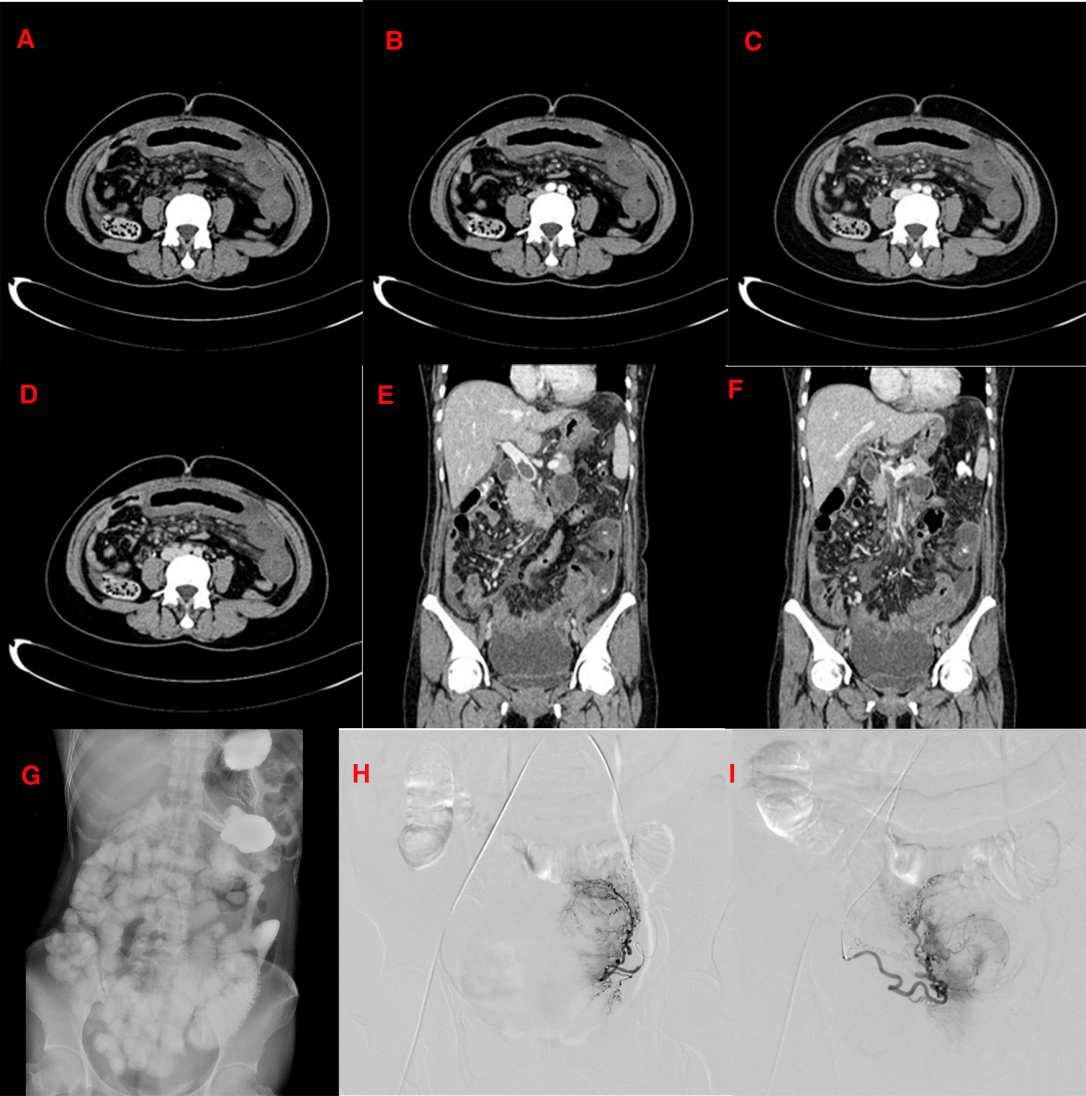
(December 15, 2016) CT scan of the patient on first admission. (A–F): The CT shows that the left middle and upper abdomen is partially empty, and the ileal bowel wall is thickened and swollen; filling defects can be seen in the lumen of the main portal vein and its branches and the superior mesenteric vein and its branches. A small amount of effusion is seen around the liver, spleen, and intestine. The enhancement of the right hepatic lobe is not uniform in the arterial phase of the enhanced scan, and the portal venous phase and the delayed phase are in isodensity. A small circular low-density shadow with a diameter of approximately 0.5 cm is seen in the IV segment of the liver near the diaphragm. There is no enhancement on the enhanced scan. The uterine shadow is enlarged, its internal density is uneven, and multiple lumpy and patchy areas of reduced density can be seen. The enhancement scan is uneven; (G): The upright abdominal plain film shows that the intestinal tube in the abdomen is slightly dilated. (H): Uterine arteriography shows that the left and right uterine arteries are thickened and disordered, and the uterus is enlarged in the parenchymal stage. (I): After uterine artery embolization, most left and right uterine arteries are blocked.
